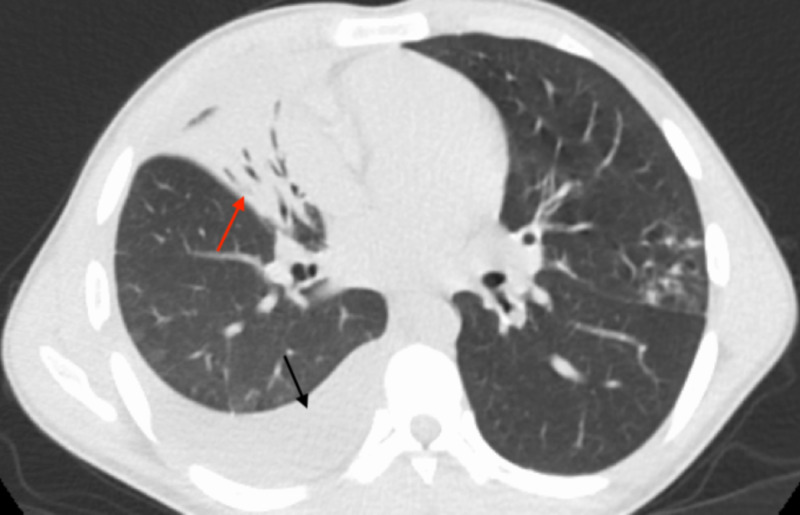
CT of the thorax demonstrating right middle lobe consolidation with air bronchograms and bronchiectasis (red arrow). Moderate-sized right pleural effusion (black arrow).
